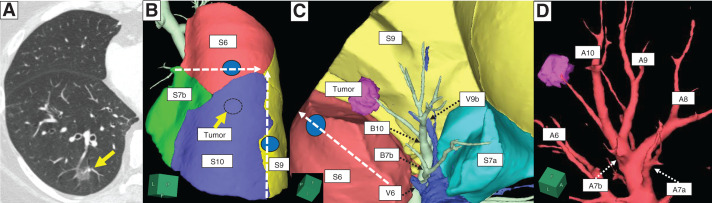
(A) CT image showing a 15-mm part-solid nodule in the right S10 segment (yellow arrow: tumor). (B) 3D reconstruction CT showed S7b interposed between S6 and S10. Preoperative bronchoscopic marking with indigo carmine was performed for B6c and B9a (2 blue spots) to guide the intersegmental plane (white dotted arrows indicate the estimated intersegmental resection lines). (C) 3D-CT imaging from the pulmonary ligament approach showing B7b positioned proximal to B10. Initial dissection of B7b enables easier access to B10. Due to imaging limitations, V6c could not be reliably reconstructed (white dotted arrow: estimated intersegmental resection line; blue spot: bronchoscopic marking for B6c; black dotted arrow: each branch of pulmonary vein and bronchus). (D) 3D-CT imaging of pulmonary arteries showing A7b originating from the A9+10, whereas A7a branches from the basal pulmonary artery. B7b, dorsal branch of B7
